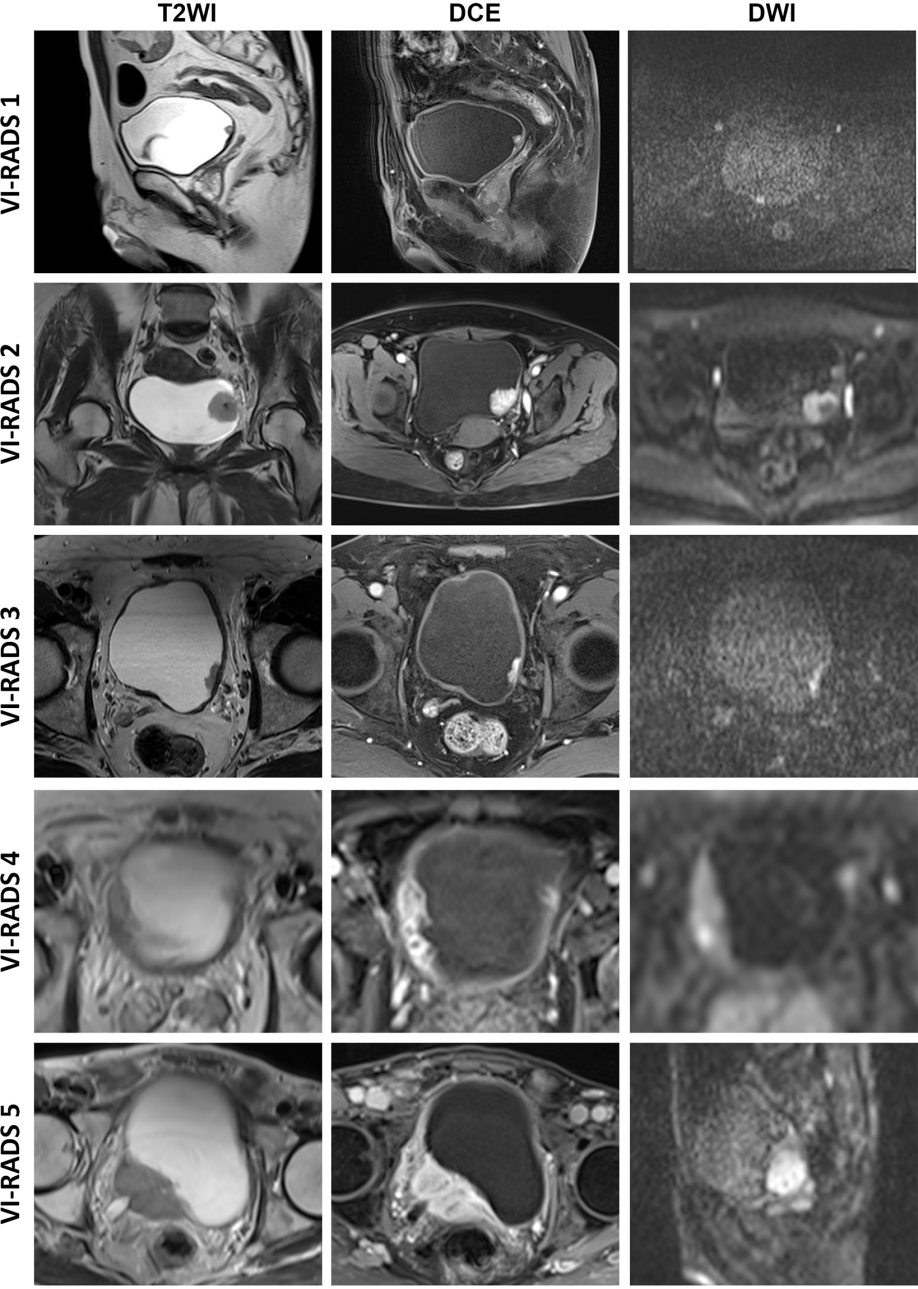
Typical images of VI-RADS scores. VI-RADS, Vesical Imaging-Reporting and Data System; DCE, dynamic contrast-enhancement; DWI, diffusion-weighted imaging.
